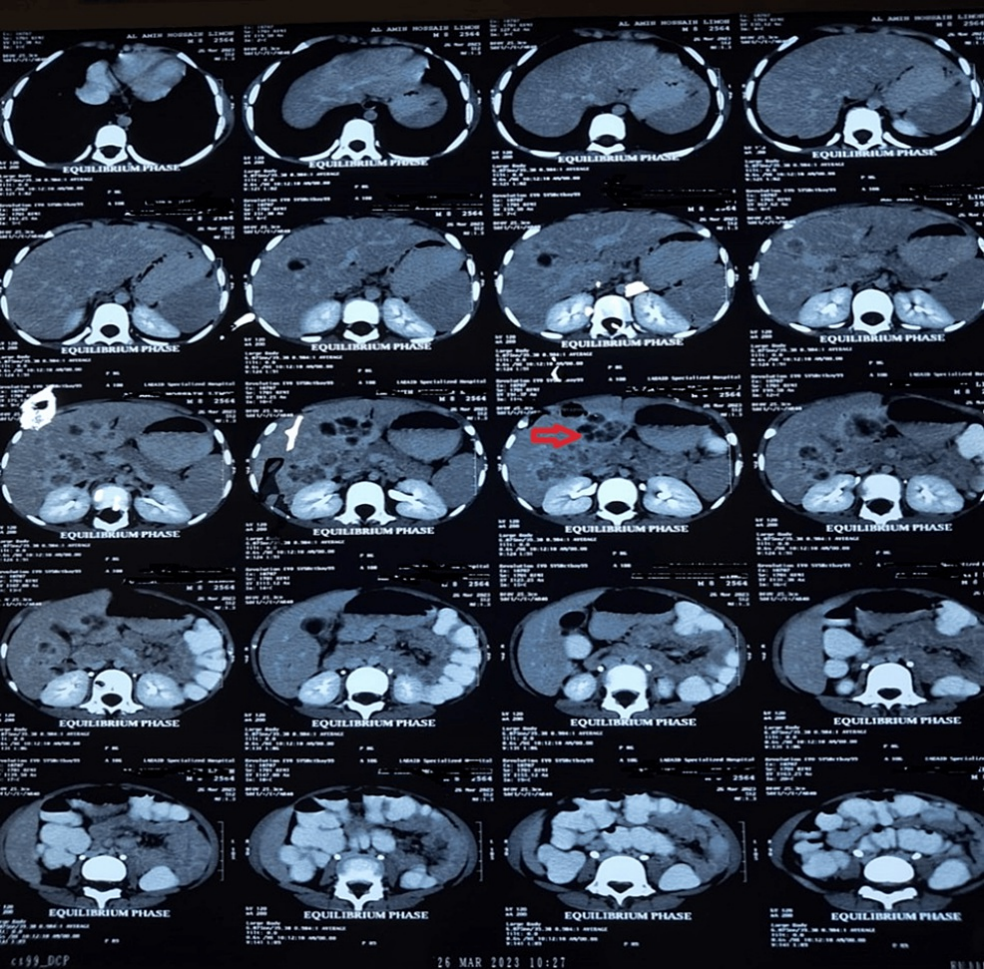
Contrast CT scan of the hepatobiliary system
